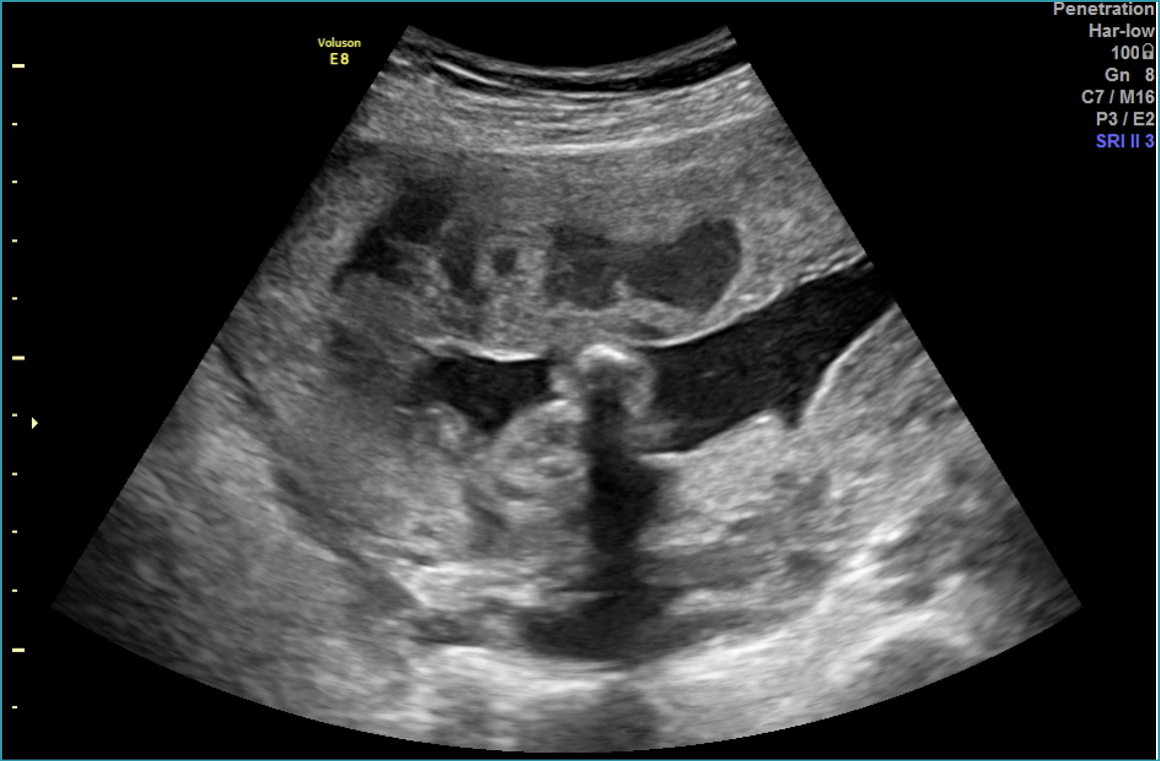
Grayscale ultrasound showing intraplacental hypoechoic images in the lower and anterior uterine segment compatible with placental lacunae in placenta previa accrete
